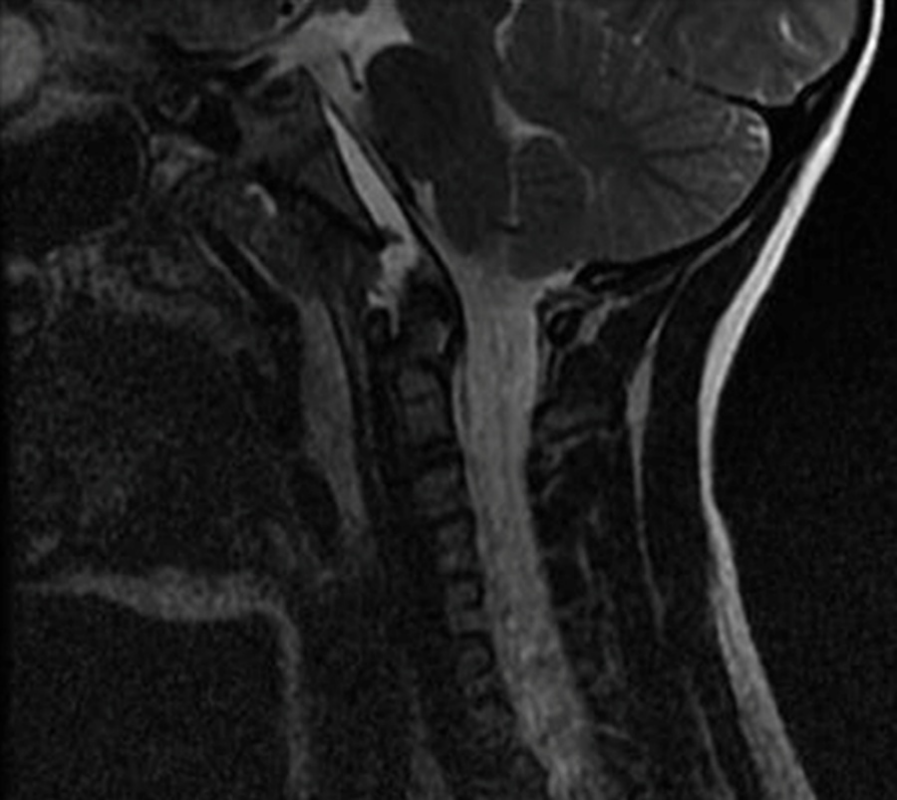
T2 weighted sagittal MRI of the cervical spine without contrast. There is hyperintesity underneath the tectorial membrane extending from C2 to the upper margin of the clivus with apparent discontinuity of the apical ligament.
